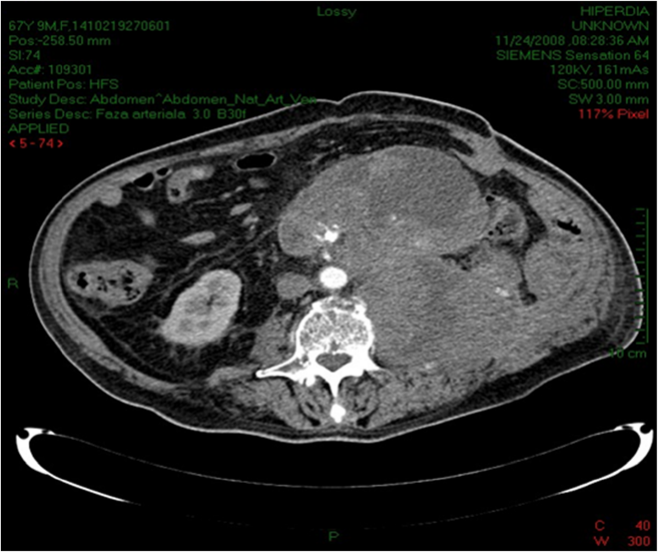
CT scan 6 months after surgery. Massive local relapse.
